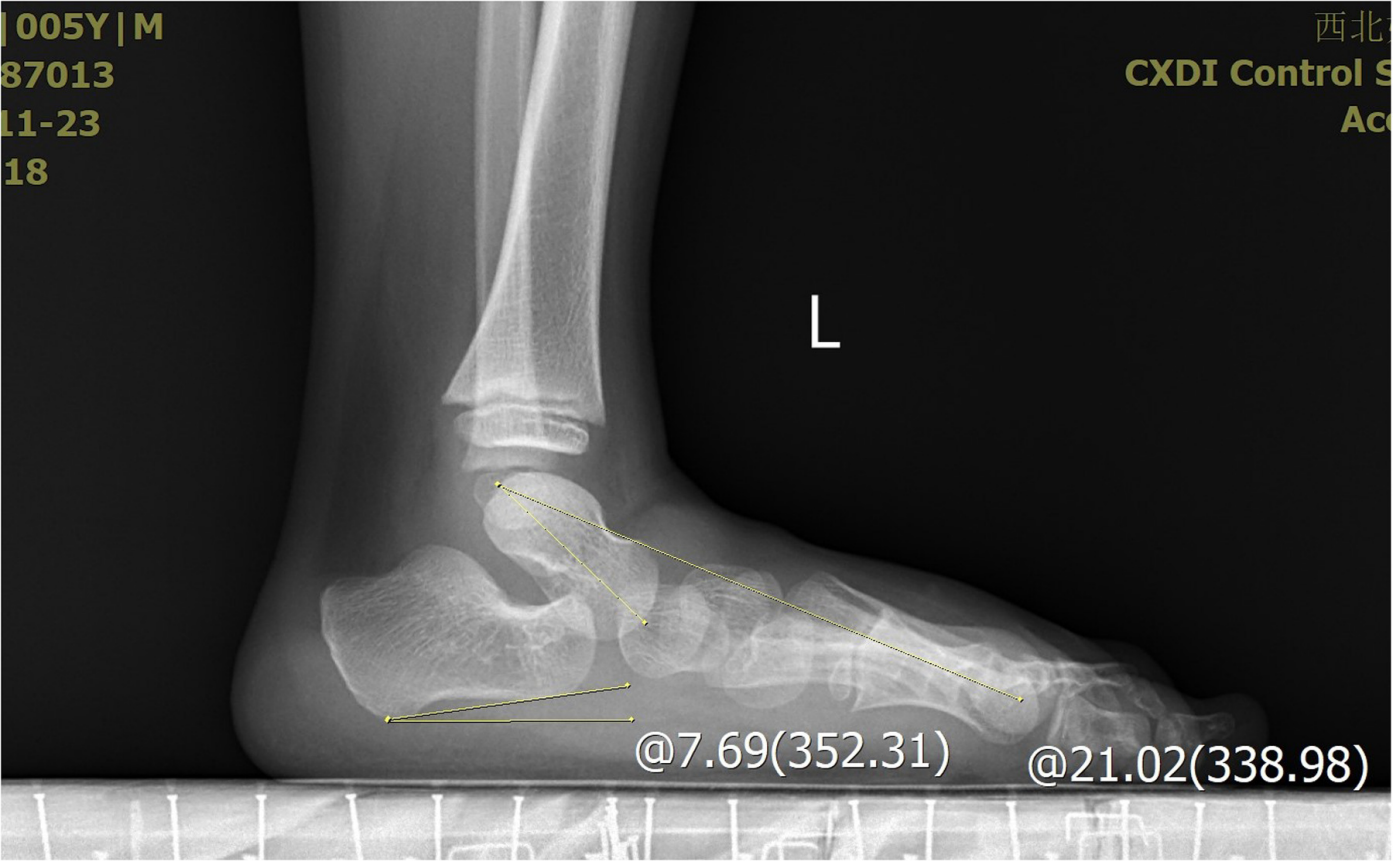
The X-ray demonstrated the measurement of the calcaneus inclination angle and Meary's angle.
